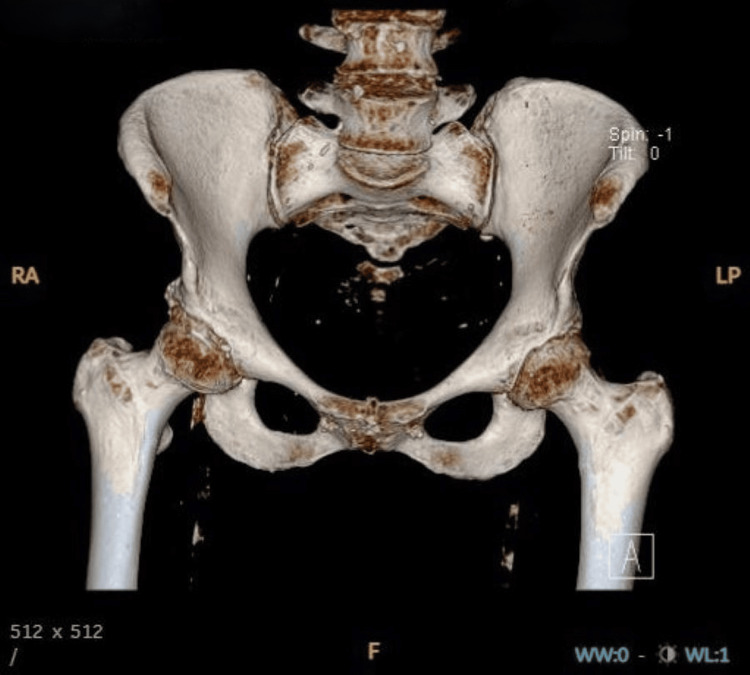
3D CT scan of the pelvis without contrast, anteroposterior view image 3D: three dimensional, CT: computed tomography
